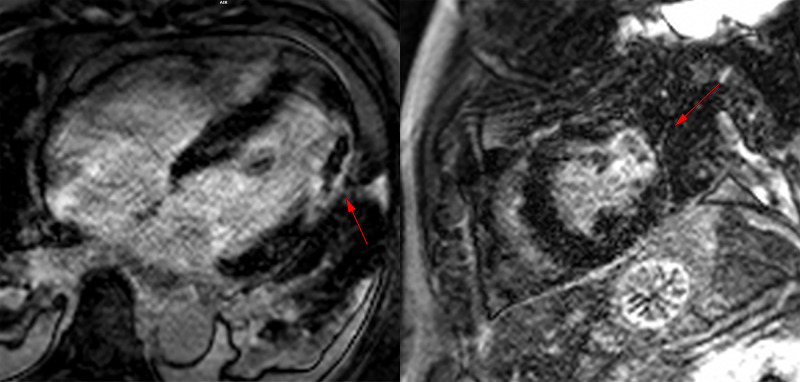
Cardiac MRI. Cardiac MRI with late gadolinium enhancement (LGE) images of the apical four chamber (left) and the short axis views (right). The LGE images showing transmural scar of the anterolateral wall as indicated by the red arrows.
